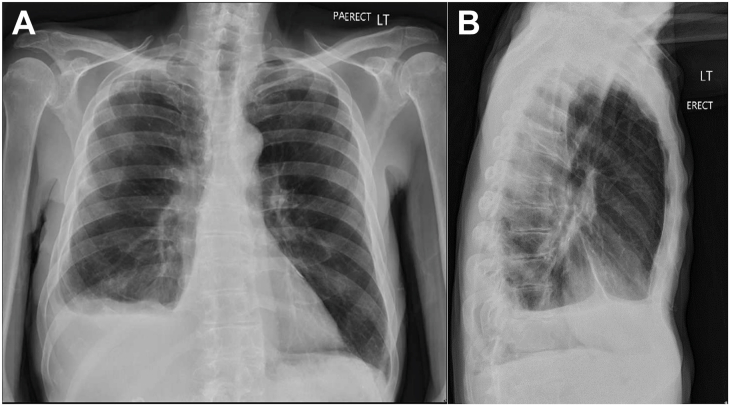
Two-view radiograph showing resolution of the right-sided urinothorax. (A) anterior view. (B) sagittal view.
